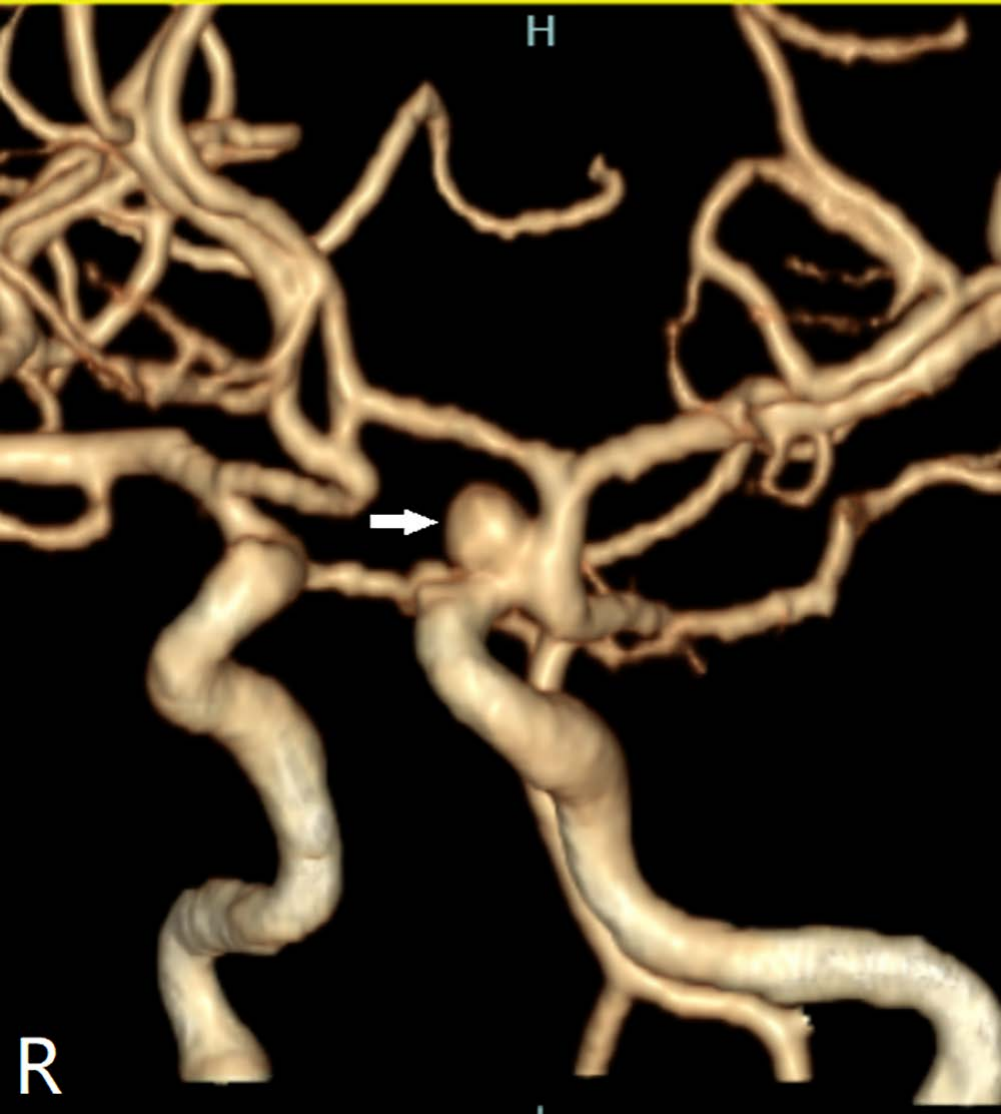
Computed tomography angiography (CTA) performed at 2 weeks post-injury showing a left internal carotid artery (ICA) ophthalmic aneurysm (arrowhead).
